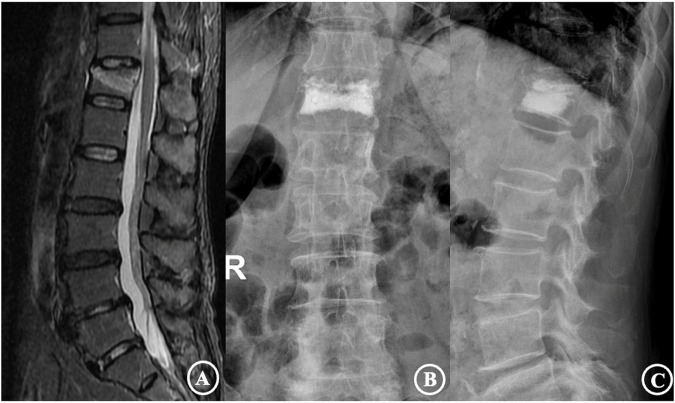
The patient was a 72-year-old female diagnosed with an osteoporotic vertebral compression fracture of T12. (A) is a preoperative MRI film suggesting a fresh vertebral compression fracture of the T12 vertebra; (B,C) are frontal and lateral X-rays after percutaneous vertebroplasty, showing a type I cement distribution and good cement dispersion.
